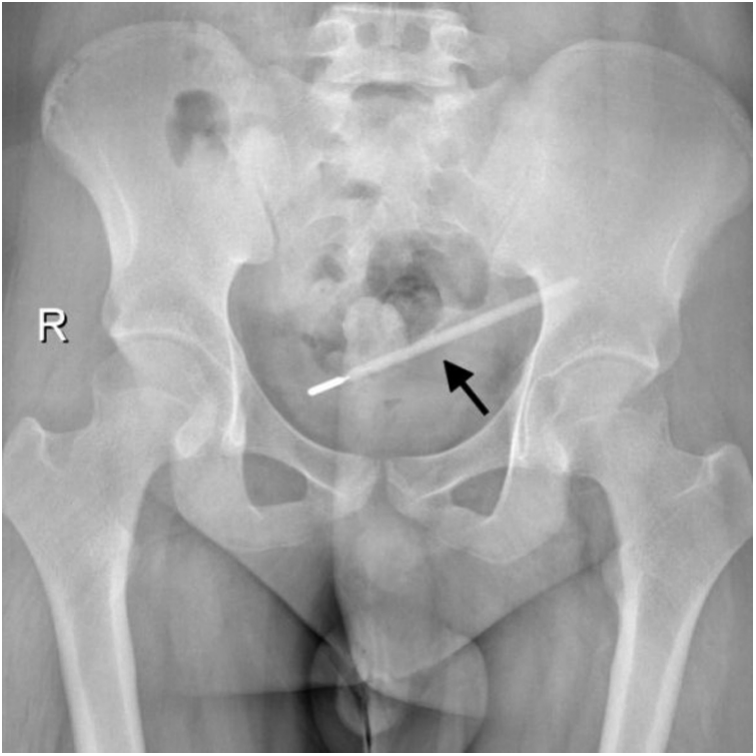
Abdominal upright x-ray showing a foreign body of a thermometer (the site pointed to by the arrow).
